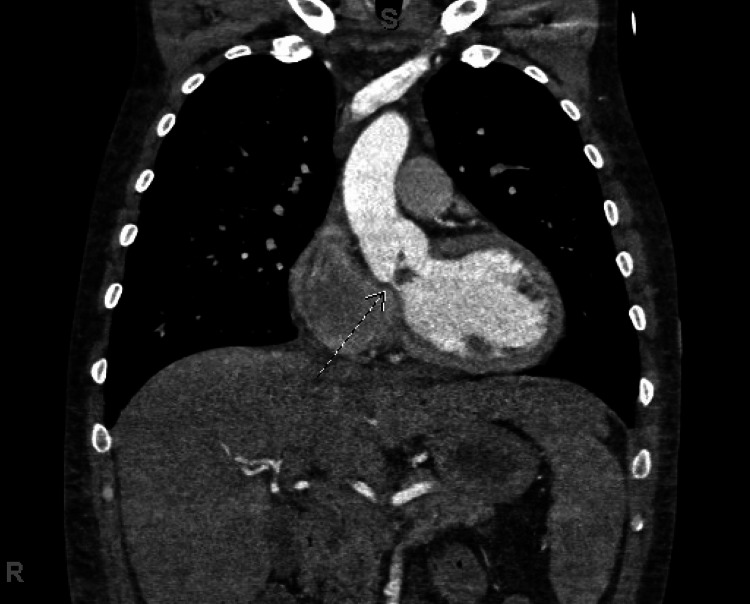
Aortic valve vegetation on computed tomography angiography of the chest
